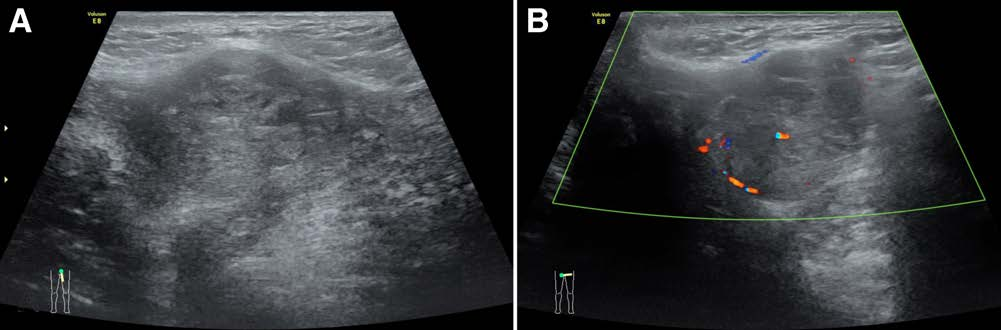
(A, B) Ultrasonography showing in homogenous echoes under the labia majora.
